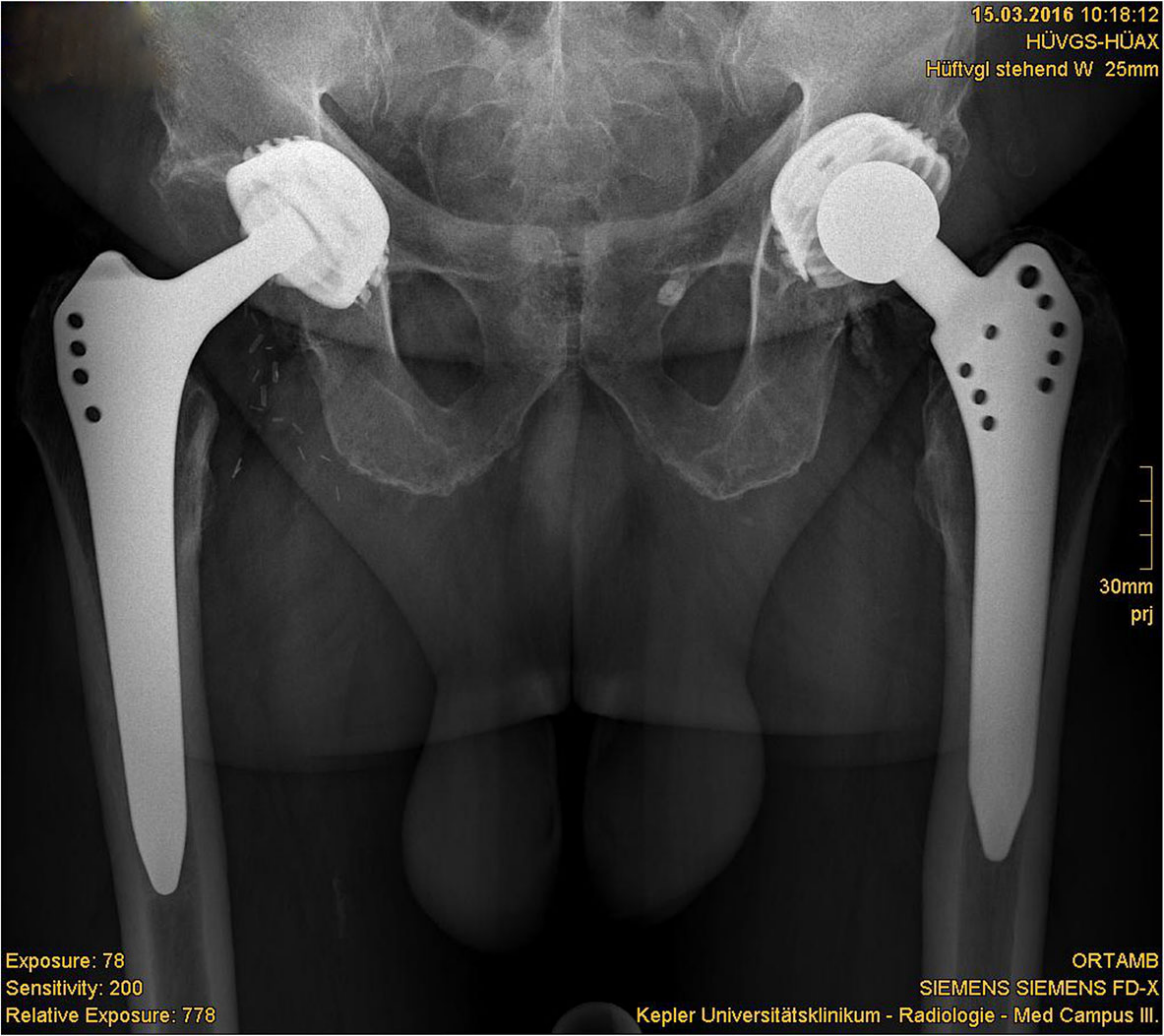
Radiograph showing excellent results after 30 years in a highly active 73-year-old male (l)
